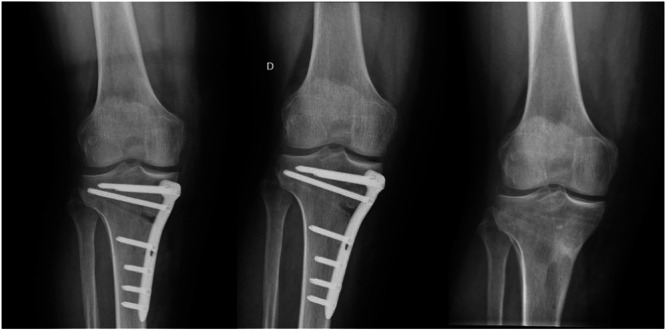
Removal of osteotomy plate in a patient with deep infection diagnosed at 84 days. In the center, a radiograph prior to removal. On the right, a radiograph three months after removal showing consolidated osteotomy.
